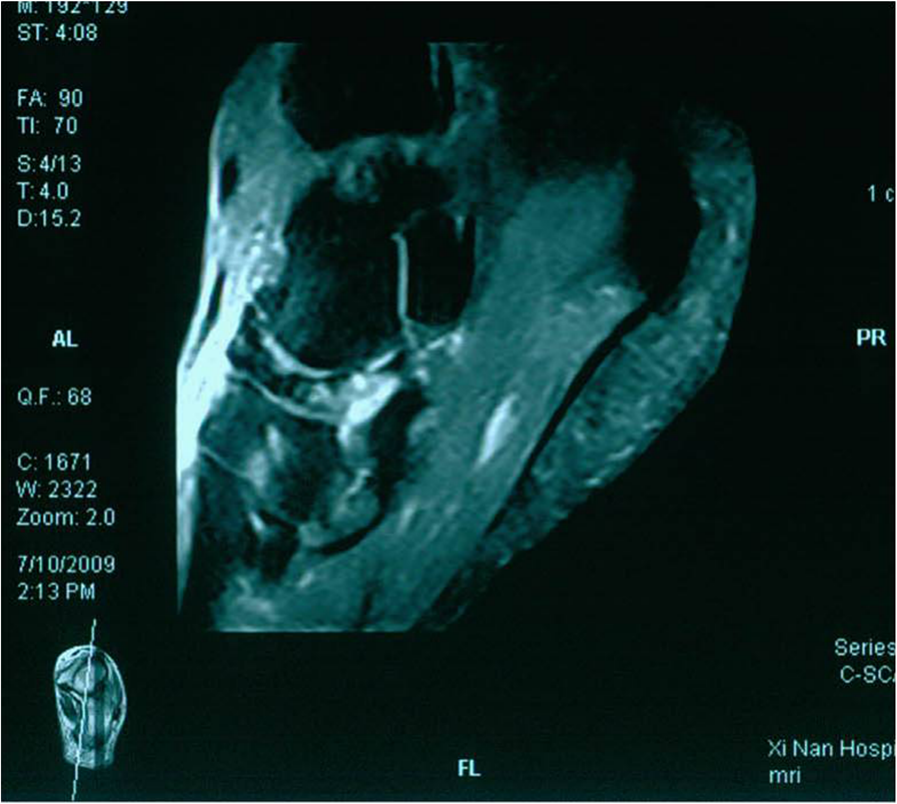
The necrosis of navicular and severe talonavicular joints arthritis in T2 fast-suppressed image of MRI scans
